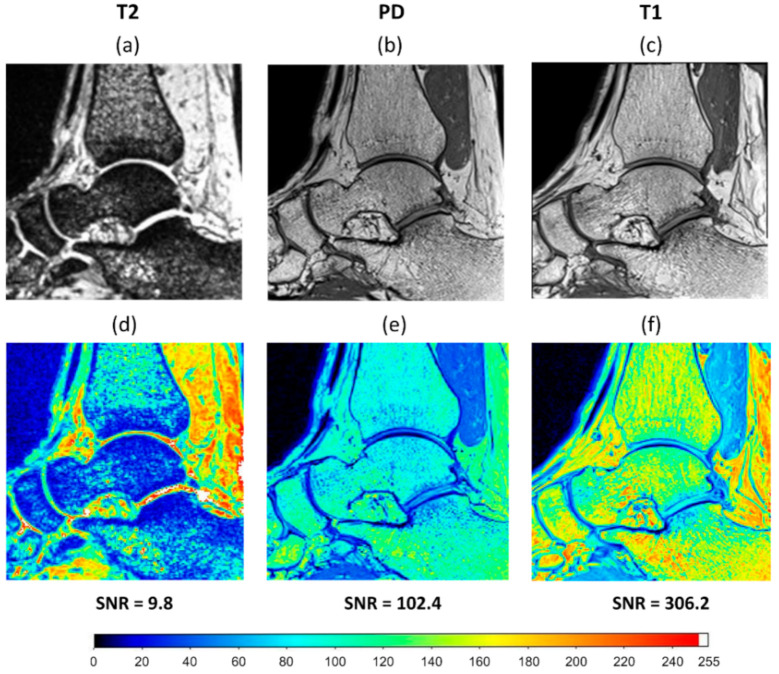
A sagittal slice of the clinical MRI of an ankle taken using three different MRI sequences: (a) T2 weighted; (b) proton density (PD) weighted; (c) T1 weighted. Signal intensity map (ranged from 0 to 255) and signal to noise ratio (SNR) achieved for (d) T2 weighted, (e) PD weighted, and (f) T1 weighted sequences.
